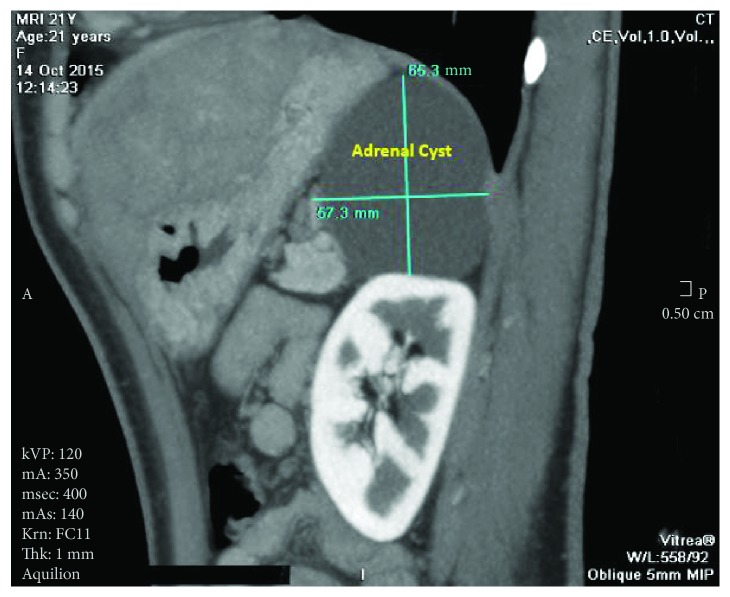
Computed tomography showing a large adrenal cyst measuring 65 × 57 mm.
